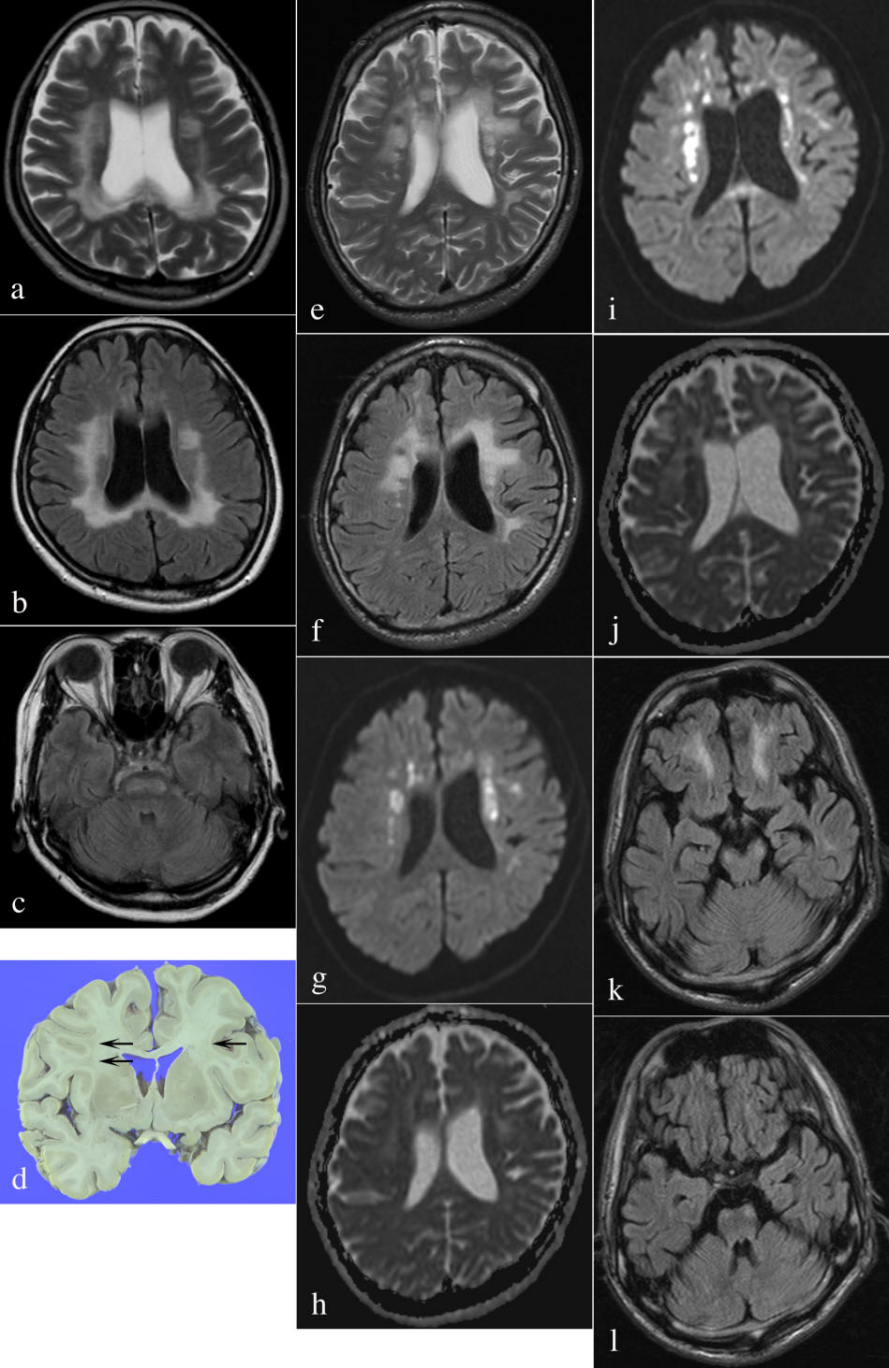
The MRI of Case 1 (a–c) shows a high-intensity signal in the cerebral white matter and corpus callosum on T2-WI (a) and FLAIR (b). This signal descends along the pyramidal tract, which is demonstrated on FLAIR in the pontine base (c). Grossly, the autopsied brain of Case 1 (d) shows bilateral, patchy discoloration of the white matter (arrows). The MRI of Case 2 (e–l) shows a high-intensity signal in the cerebral white matter on T2-WI (e) and FLAIR (f). This signal is partially high on DWI (g) and low on ADC (h), which is reminiscent of acute infarction. The follow-up MRI about 1 year after the onset shows persistence of the previously recognized signal, which is still high on DWI (i) and low on ADC (j). In addition, a previously unrecognized abnormal signal is apparent along the pyramidal tract, which is shown on FLAIR in the cerebral peduncle (k) and pontine base (l). MRI, magnetic resonance imaging; T2-WI, T2-weighted image; FLAIR, fluid attenuated inversion recovery; DWI, diffusion weighted image; ADC, apparent diffusion coefficient map.
